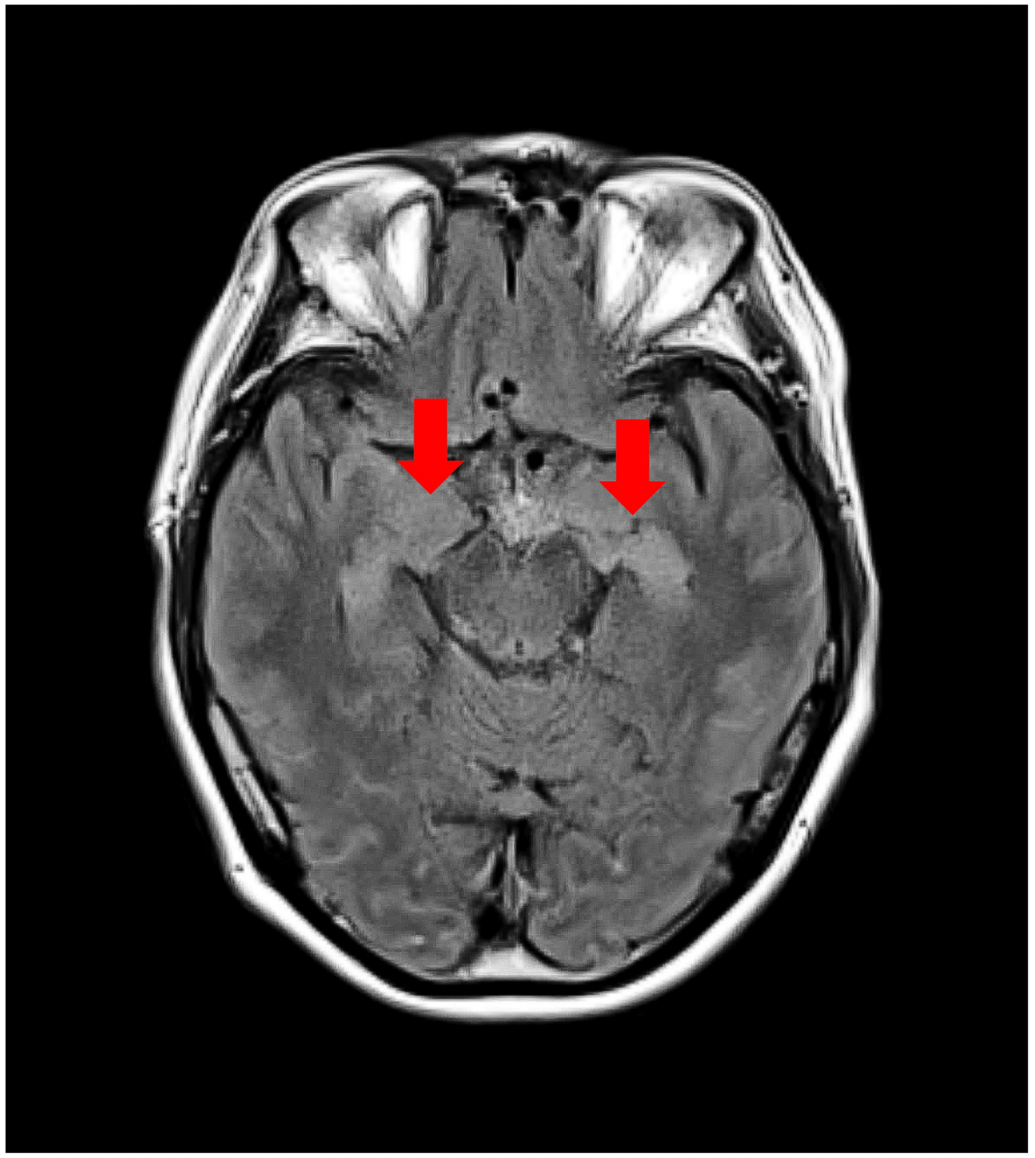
MRI (FLAIR) showed hyperintensities in both temporal lobes (red arrow) FLAIR: fluid-attenuated inversion recovery
